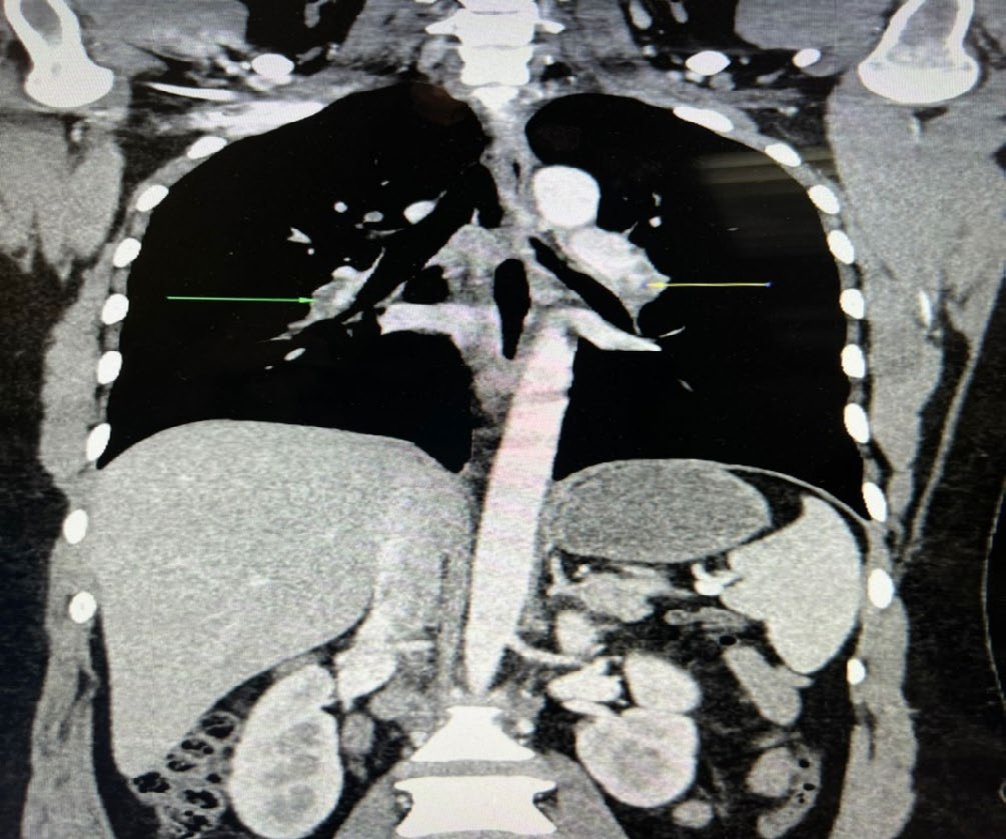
Shows a high‐resolution CT (HRCT) scan of the chest, revealing patchy areas of ground‐glass opacities in the right upper and middle lobes.
